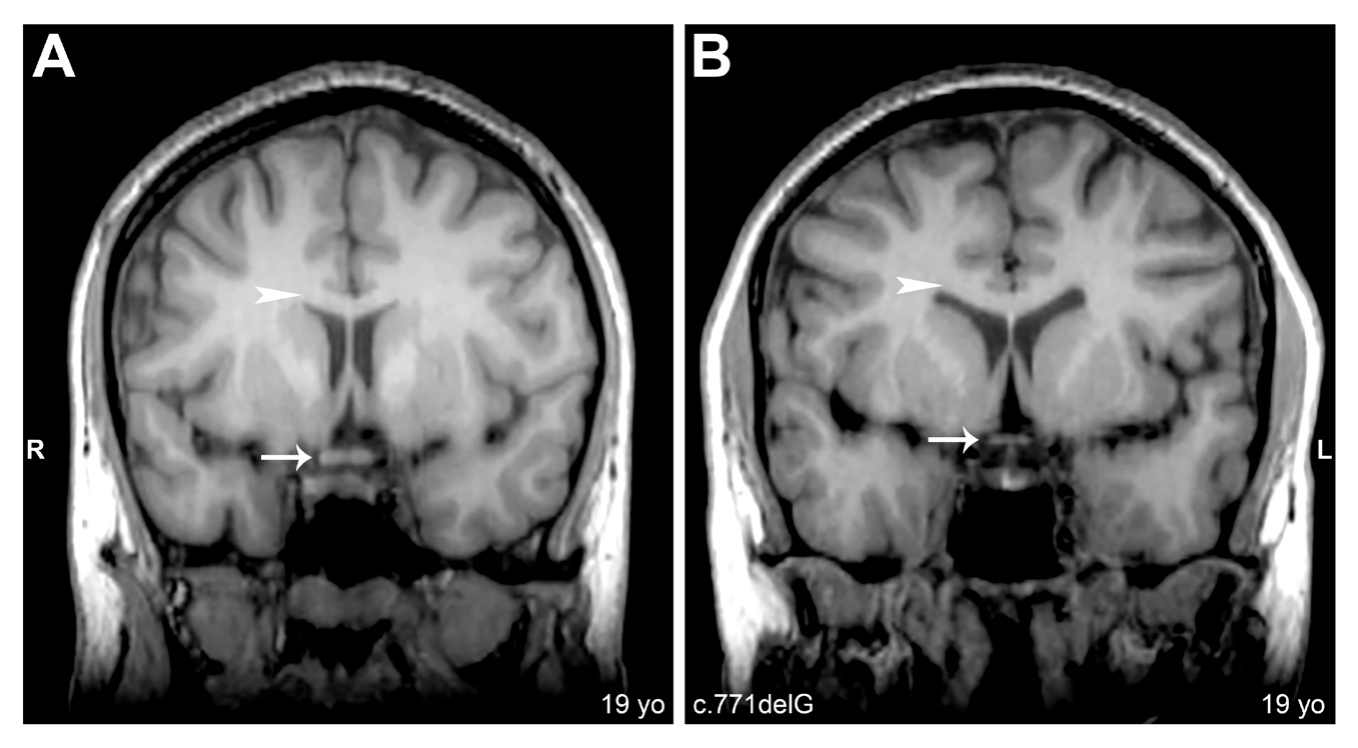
Optic chiasm and corpus callosum: Coronal cerebral T1-weighted magnetic resonance images, slice thickness 1.2mm. (
